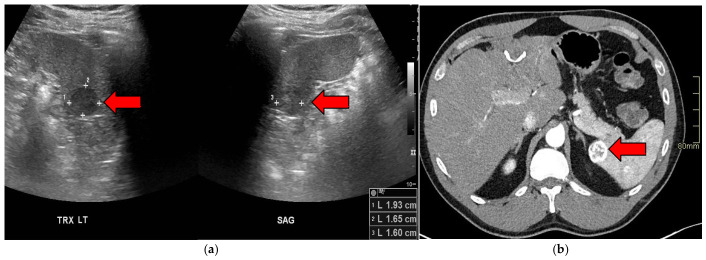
49-year-old male with *Echinococcus multilocularis*. (a) Ultrasound shows a well-circumscribed hypo-echoic lesion with daughter cysts. (b) On axial contrast-enhanced CT, there are multiple well-defined hypodense cystic lesions with peripheral and septal calcifications.
